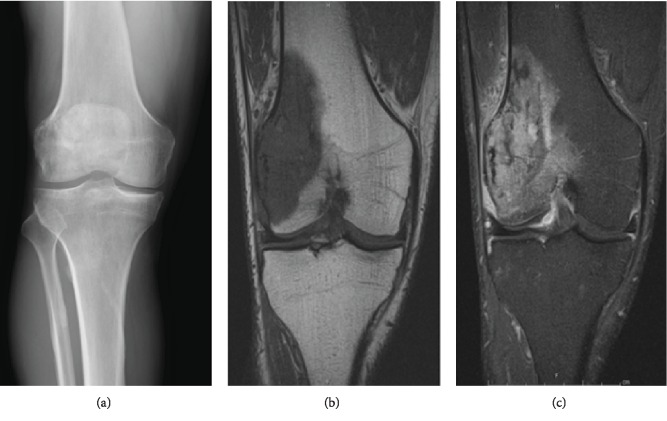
(a) X-ray of the involved distal femur shows a mixed lucent sclerotic lesion with permeative borders involving the lateral distal femoral metaphysis. (b) MRI shows a bone lesion replacing the marrow in the LFC and distal metadiaphyseal junction with dark heterogeneous signal on T1W and brighter signal intensity on T2W sequences. (c) Perilesional edema is seen on the T2W sequences both in the intraosseous and periosteal regions. There was no definite associated STM. Maximal dimensions were just under 8 cm.
